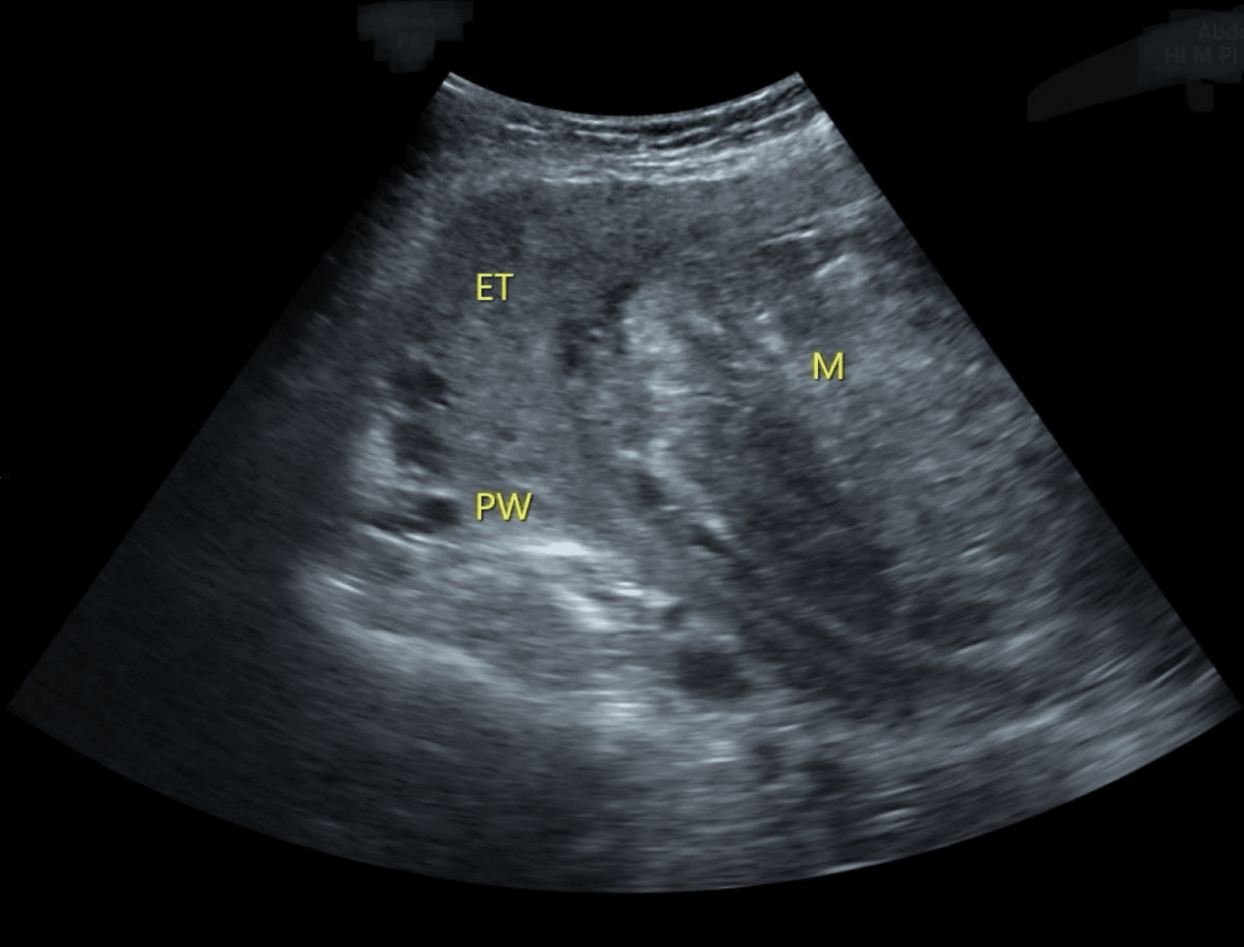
Ultrasound image showing huge fundal fibroid arising from the anterior wall of the uterus M: Mass; PW: Posterior wall of the uterus; ET: Endometrial thickness
